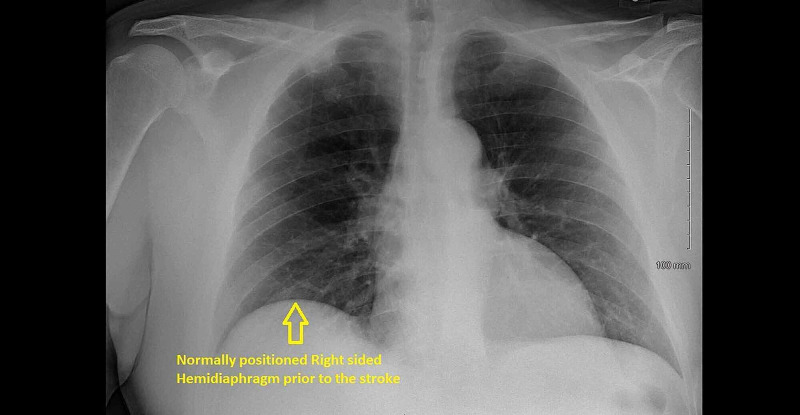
Anteroposterior chest X-ray depicting a normally positioned right-sided hemidiaphragm prior to the stroke.
